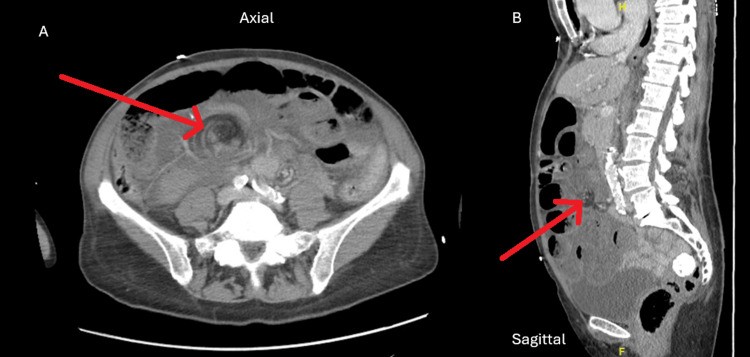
CT findings demonstrating a positive swirl sign with obstructive findings including a dilated small bowel. The red arrow demonstrates the swirl sign. A: Axial plane. B: Sagittal plane.
